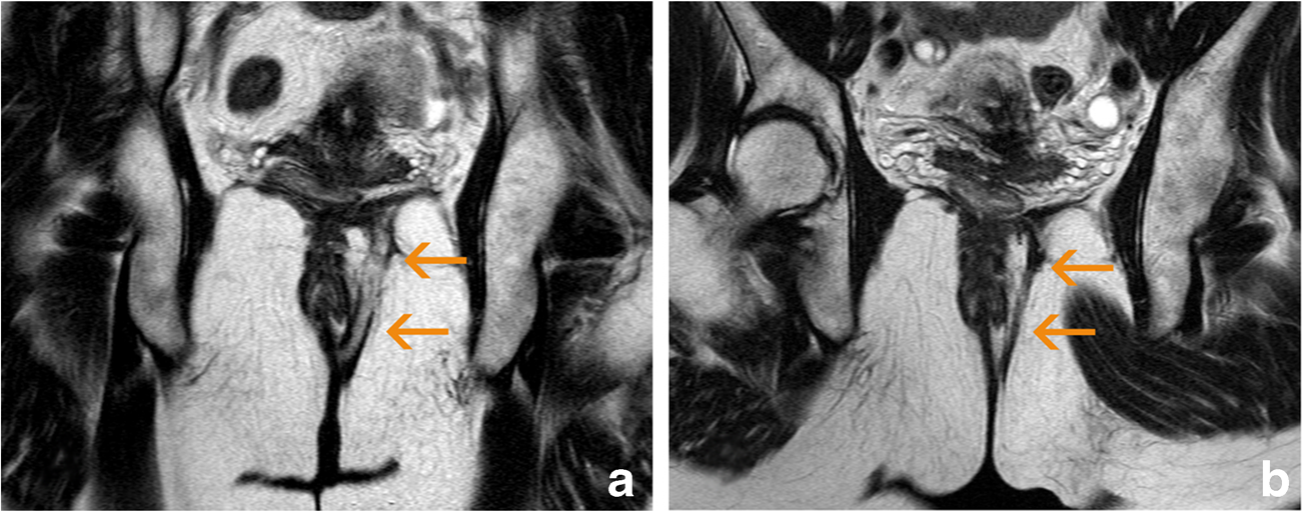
Complete response after anti-TNF therapy. a Axial T2-weighted sequences at baseline show a complex left trans-sphincteric fistula with hyperintense paths (arrow); b Axial T2-weighted sequences after 24 weeks of treatment show that all paths have decreased in thickness and exhibit hypointensity (arrow)
